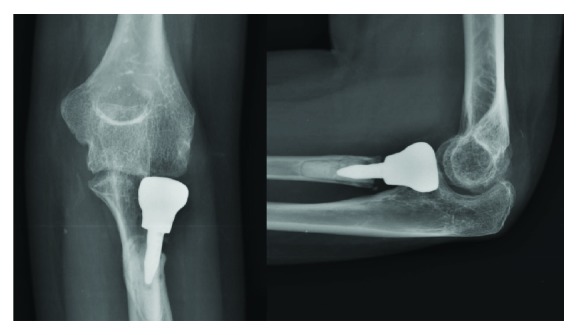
Postoperative plain radiographs at the last follow-up.
